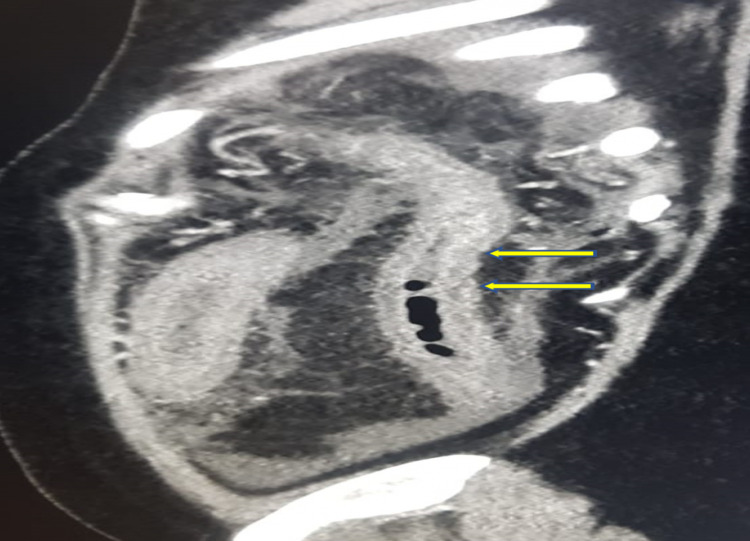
Computed tomography, sagittal view, showing decreased mural enhancement with mural thickening and luminal narrowing of the descending colon (yellow arrows).
